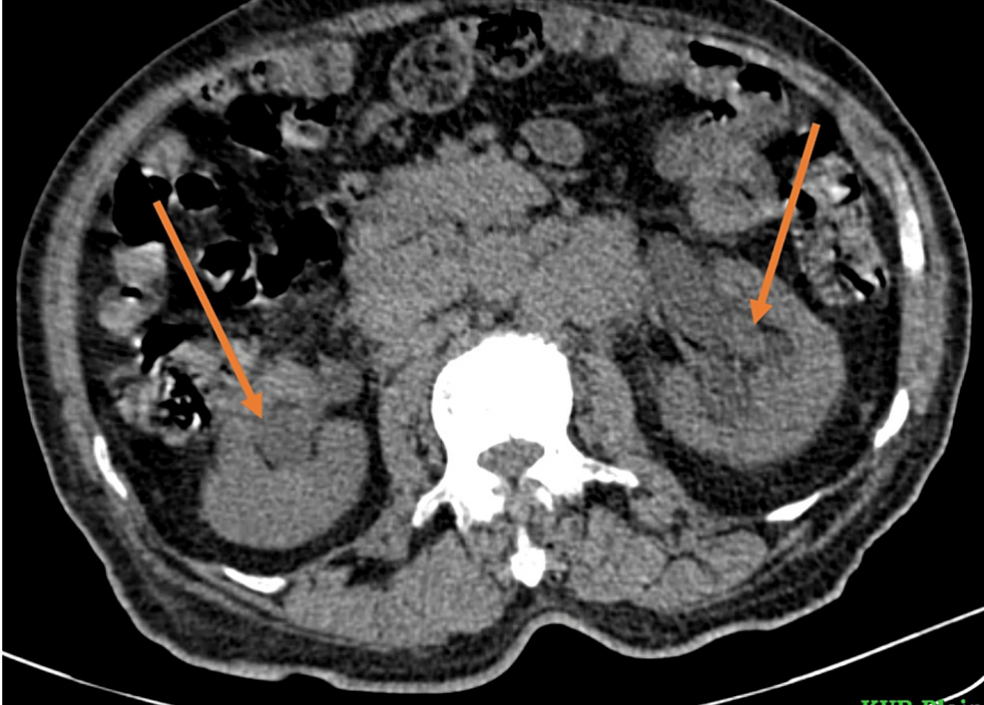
Transverse section of computed tomography of kidney, ureter, bladder indicating bilateral hydronephrosis (Orange arrows).
